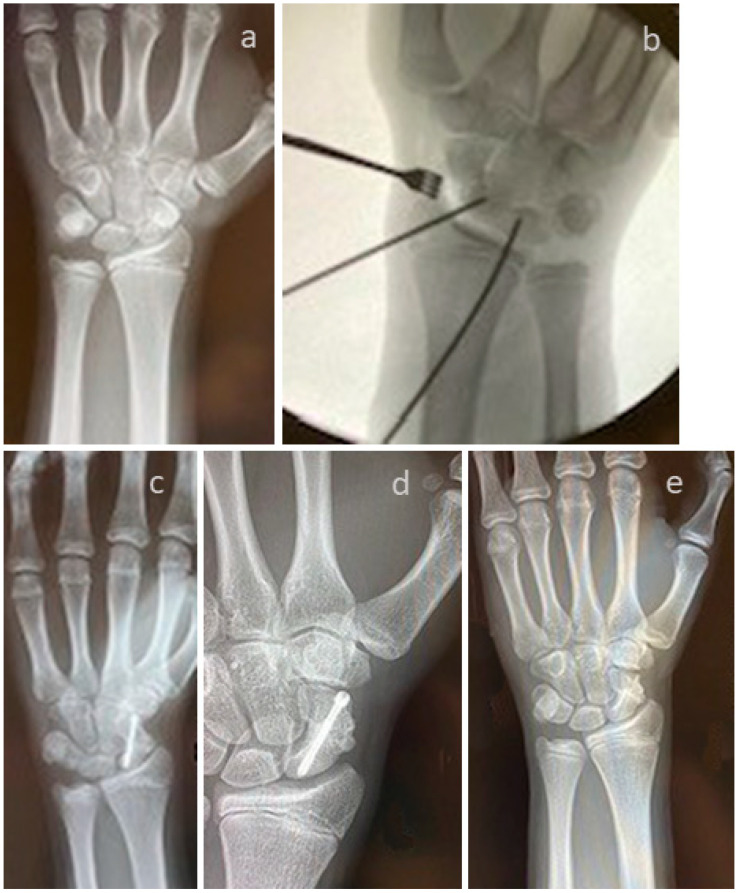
The case of a non-union of a scaphoid fracture with conservative treatment. (a): Pre-surgical X-ray; (b): interoperative fluoroscopy. Revision was performed using the Linscheid maneuver, with correction of the Humpback deformity, using an autologous iliac crest cortical bone span and a Herbert Screw. (c): X-ray two months after revision; (d): union was observed six months after surgery. Metal hardware was removed one year later. (e): X-ray 18 months post revision.
